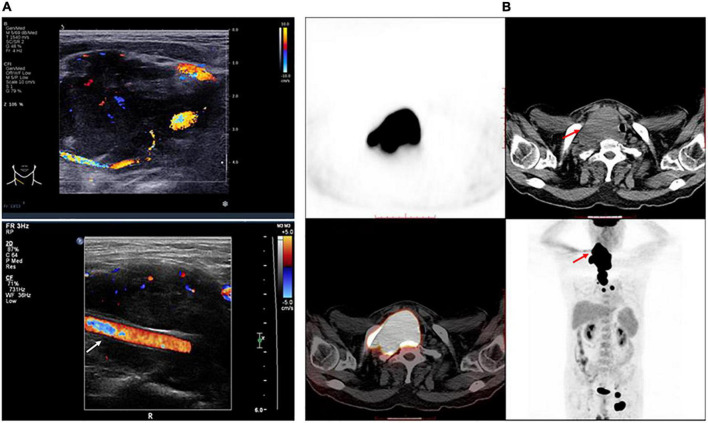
Features of the tumor. (A) Right neck ultrasonography shows a hypo-echoic region with abundant blood flow surrounding the right common carotid artery, which has normal internal blood flow. White arrow indicates the right common carotid artery. (B) The trunk positron emission tomography/computed tomography shows a large mass in the right neck surrounding the adjacent large vessels, which had a non-uniform increase in radioactive uptake. Red arrow indicates the tumor.
